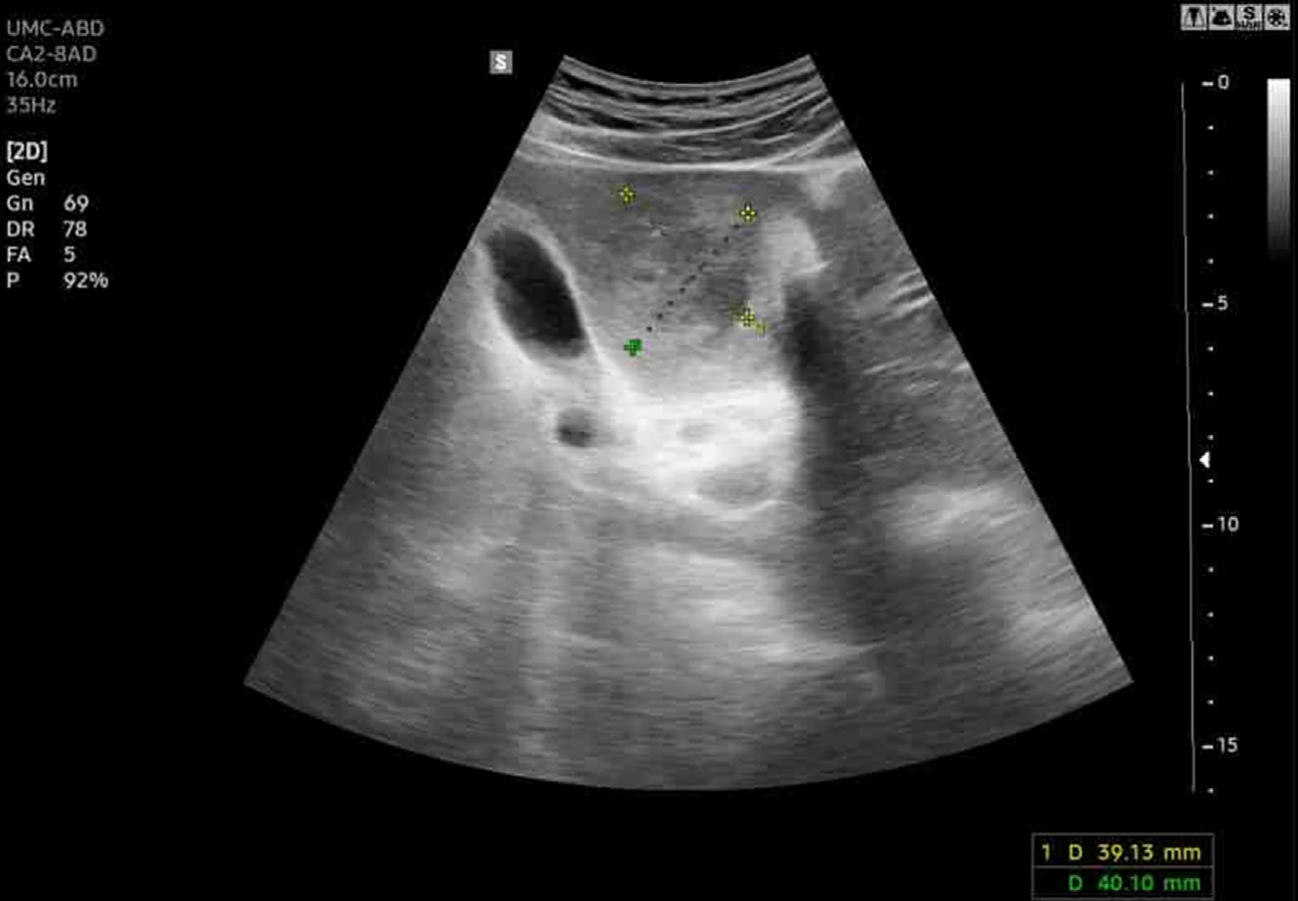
Ultrasonography shows a poorly demarcated, heterogeneous lesion in segment 4B of the liver.
